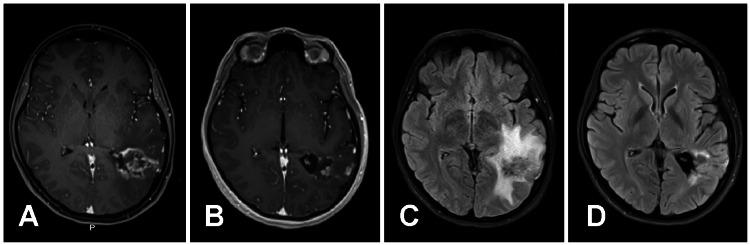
Pretreatment and Posttreatment MRI Scans of the Brain in a Patient with an Arteriovenous Malformation (A) Pretreatment MRI scan of the brain. Axial T1-weighted image obtained following IV gadolinium administration revealing prominent nodular subependymal and parenchymal enhancement, manifest as T1 shortening at the site of stereotactic radiosurgery for treatment of a left temporal lobe arteriovenous malformation (AVM). This image demonstrates the characteristic "cut-green pepper" pattern of enhancement, with an outer edge of linear enhancement within the field of radiotherapy outlining internal hazy striated enhancement. (B) Posttreatment MRI scan of the brain. Axial T1-weighted image obtained following IV gadolinium administration revealing subtle residual nodular subependymal enhancement, markedly improved compared with pretreatment scan. (C) Pretreatment MRI scan of the brain. Axial Fluid-attenuated inversion recovery (FLAIR) image at the level of treatment, trigone of the left lateral ventricle.  This image reveals prominent confluent left temporal white matter edema, manifest as T2 prolongation. This is located at the site of radiation therapy and represents vasogenic edema secondary to radiation necrosis. (D) Posttreatment MRI scan of the brain. Axial FLAIR image revealing subtle residual T2 prolongation, markedly improved compared with pretreatment scan.
